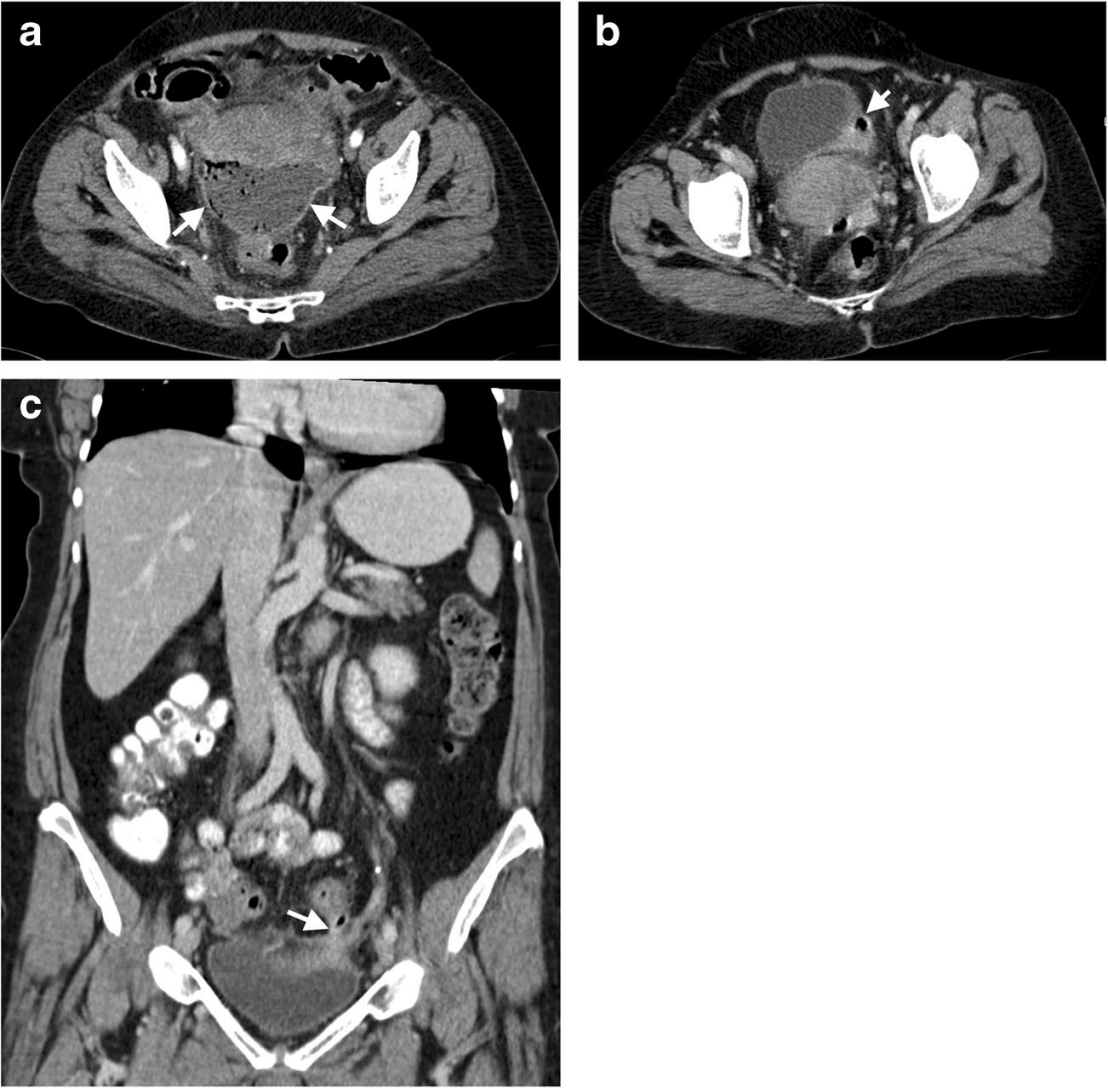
Colovesical fistula in a 46-year-old male after diverticulitis. a Axial contrast-enhanced CT of a 62-year-old woman reveals a fluid collection (*arrows*) in the Douglas pouch with air content and peripherally enhancing wall. b Contrast-enhanced CT reveals thickening of left bladder wall (*arrow*) adjacent to the diverticulum. c Coronal contrast-enhanced CT performed 5 months after first CT demonstrates a fistula (*arrow*) between the sigmoid colon and the bladder
